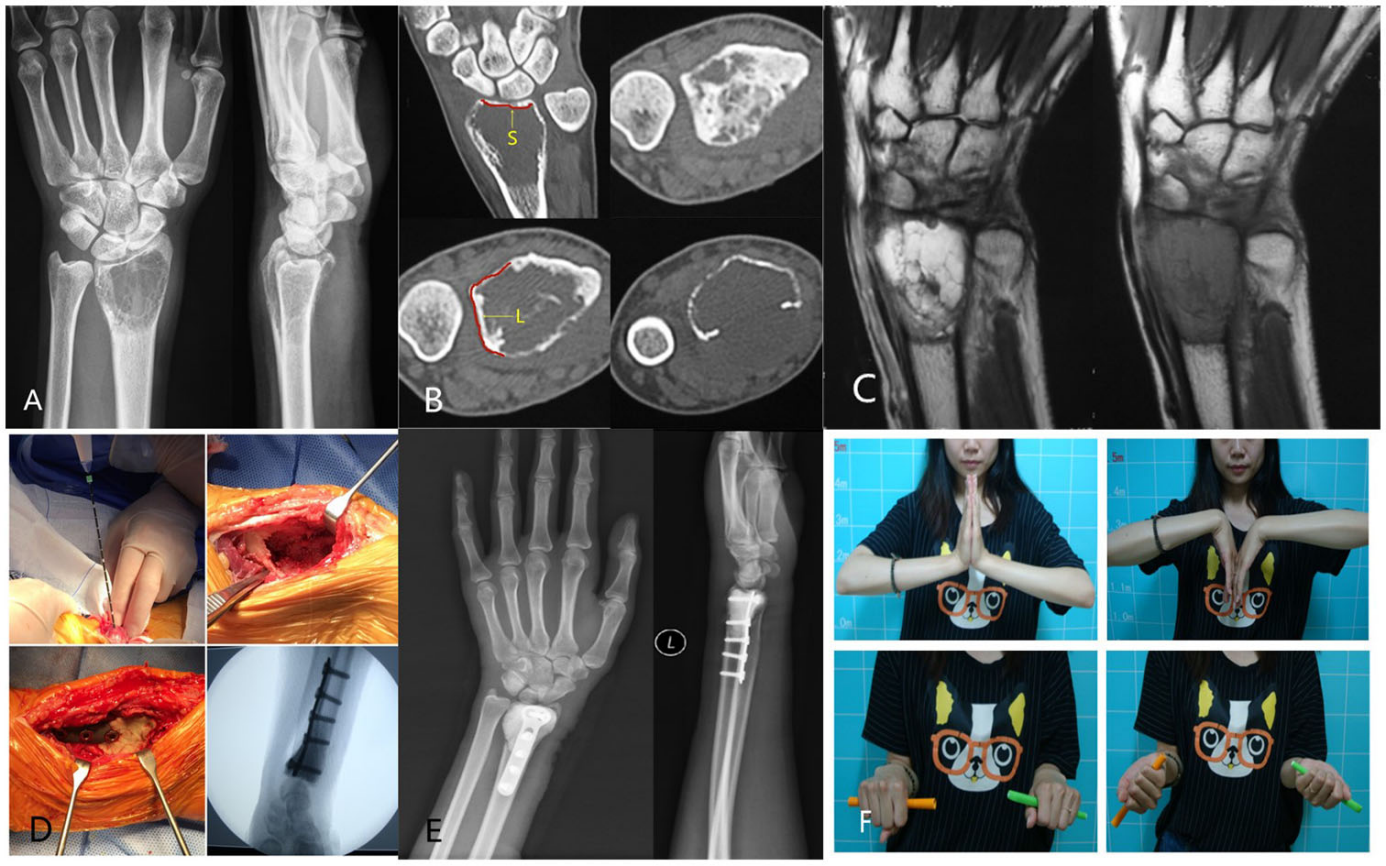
(A) Preoperative radiographs displayed expansive bone destruction at the distal end of the left radius with discontinuous bone cortex. (B) Preoperative CT showed cortical perforation on the palmar side of the radius, soft tissue extension, and no involvement of the radiocarpal joint surface. The uninvolved radiocarpal joint surface (yellow S).The DRUJ is not affected(yellow L). (C) Preoperative MRI evaluation of soft tissue extension can be completely removed. (D) Intraoperative microwave ablation combined with curettage and internal fixation with bone cement were used for treatment. (E) After 36 months of follow-up, the X-ray film showed that the internal fixation was in good position, and there was no local recurrence. (F) After 36 months of follow-up, wrist mobility was good.
